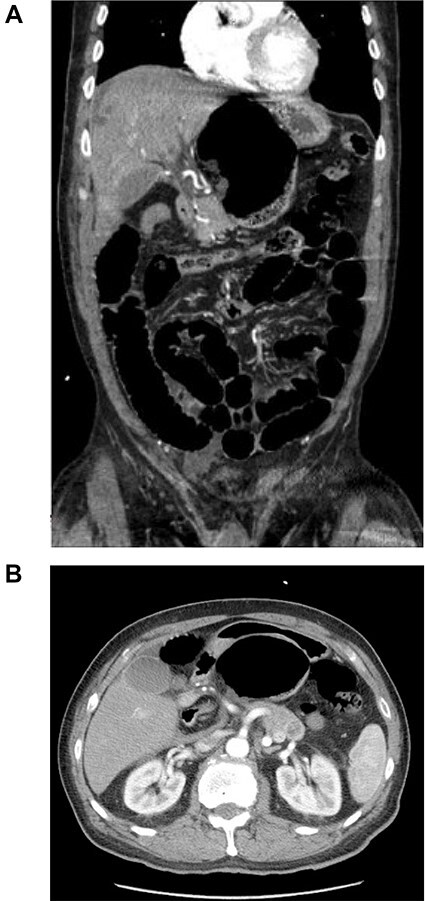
CT scan of the abdomen and pelvis with IV contrast. (A) Coronal view shows internal herniation of the cecum through the foramen of Winslow. (B) Axial view demonstrates the same findings with the stomach displaced both laterally and anteriorly by the cecum. Of note there was 1.5-cm ring-enhancing lesion located in the body of the pancreas found incidentally.
